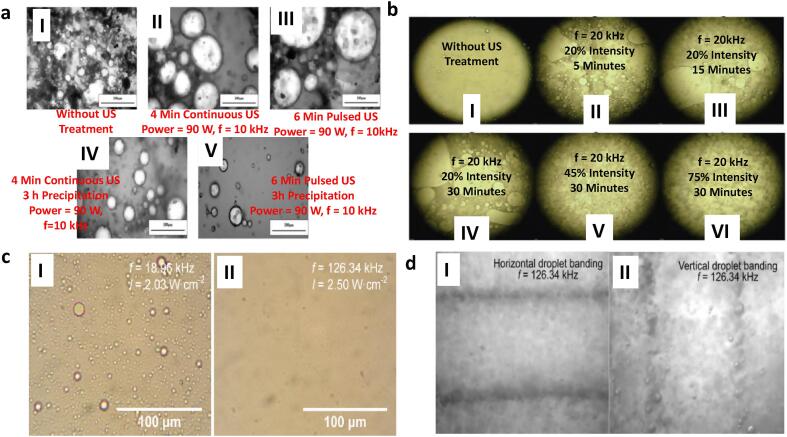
Effect of ultrasonic transducer parameter on the mechanism of demulsification: a Pulsed vs Continuous US [48]b Creaming effect under different US intensities and time [19]c Low vs High Frequency [36]d Horizontal vs Vertical Banding [36].
